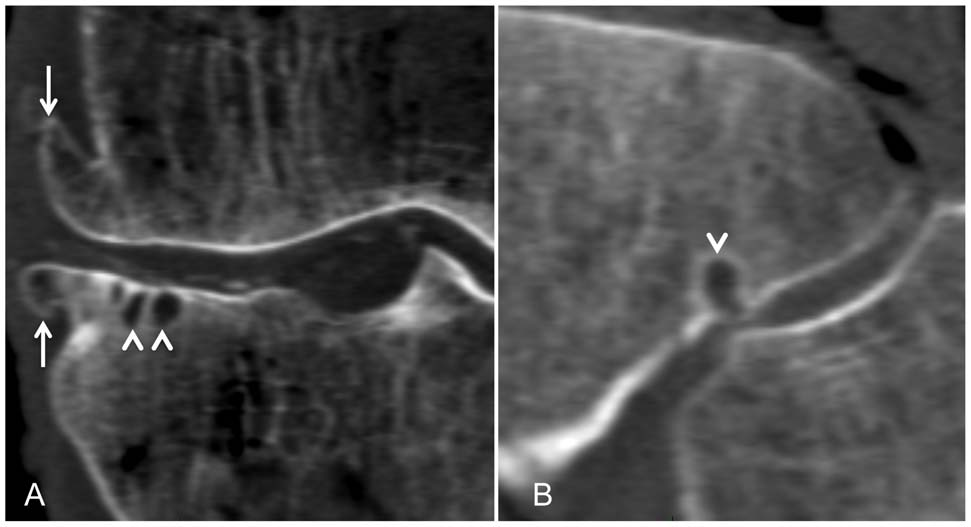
Computed tomographic scans of the same knee showing osteoarthritis in the medial femoro-tibial compartment on a coronal reformation (A) and in the proximal tibio-fibular joint on a sagittal reformation (B). Osteoarthritic changes are consistent with multiple osteophytes (white arrows) and subchondral cysts (arrow heads). Articular calcifications are visible in the femoro-tibial compartment.
